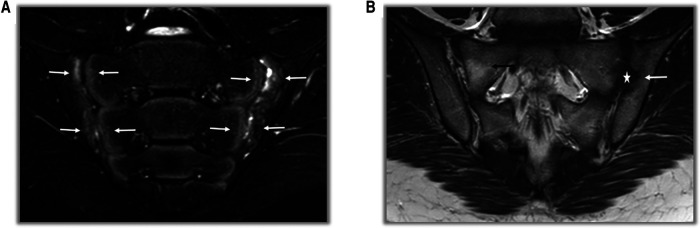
MRI findings in SpA patients. (A) On T2-weighted fat-suppressed coronal sections, areas of bone marrow edema consistent with acute sacroiliitis are observed on the facing sides of the bilateral sacroiliac joint (white arrows). (B) On T1-weighted fat-free coronal sections, erosion and irregularities at the level of the left sacroiliac joint (white arrow) and pseudo-widening (star) of the joint space are observed. In the right sacroiliac joint, there is an appearance of fat replacement (black arrow) in the sacral region.
